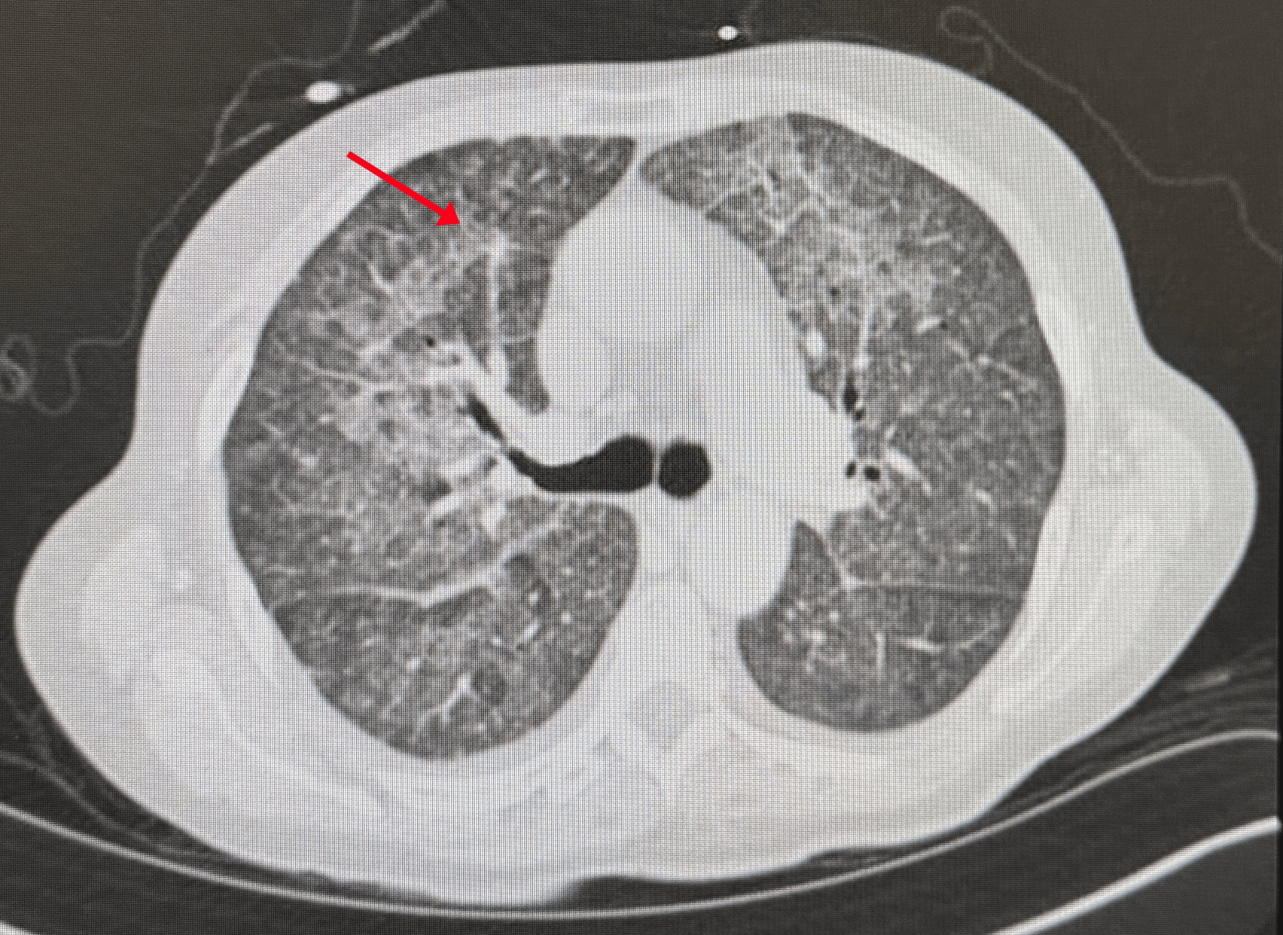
Chest CT revealing extensive ground-glass opacities with interlobular septal thickening (red arrow).
